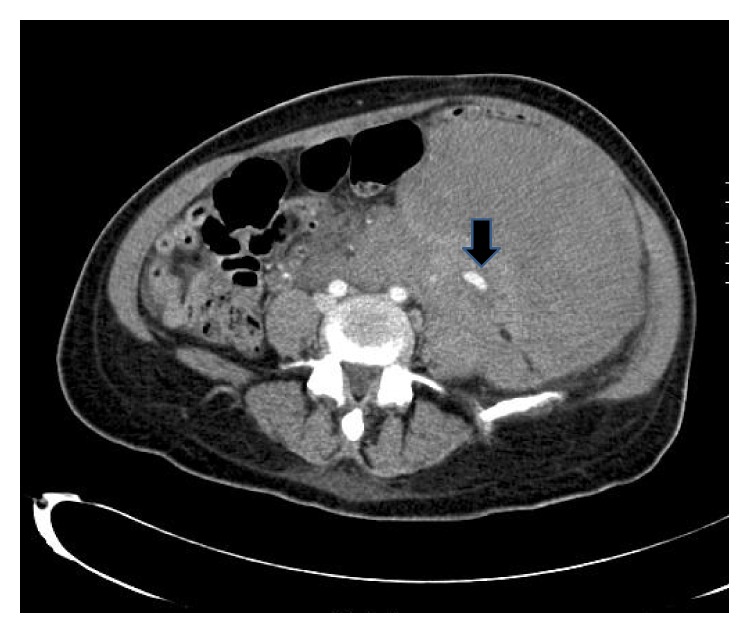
CT axial image with IV contrast: CT axial image of lower abdomen demonstrates 13 mm × 70 mm aneurysm in the left lower quadrant associated with a large hematoma. This corresponds to the aneurysm in the selective left ovarian arteriogram in Figure 3 (arrow).
